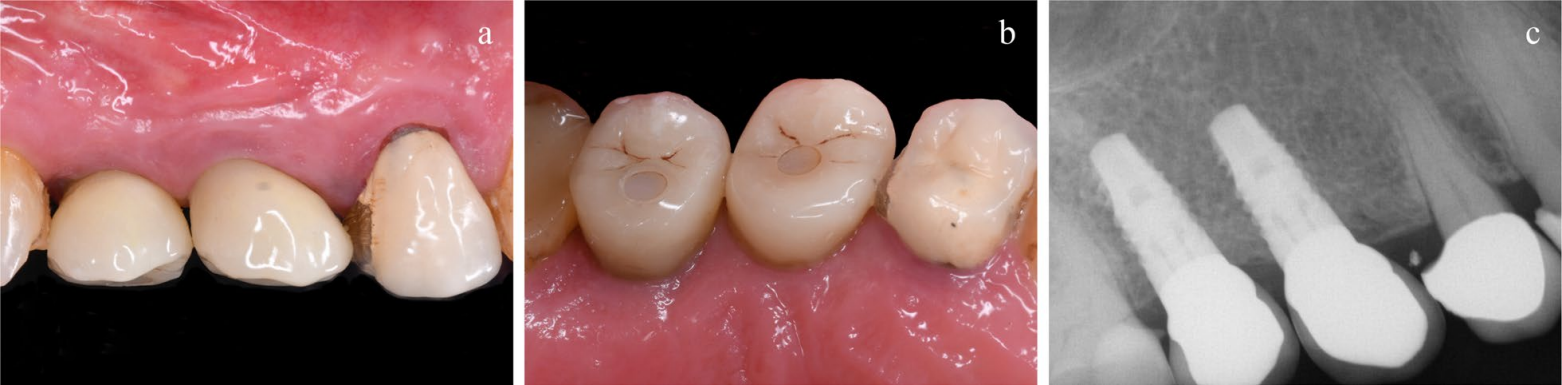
a-c: a and b clinical and c radiographic images at 5 years after placement
